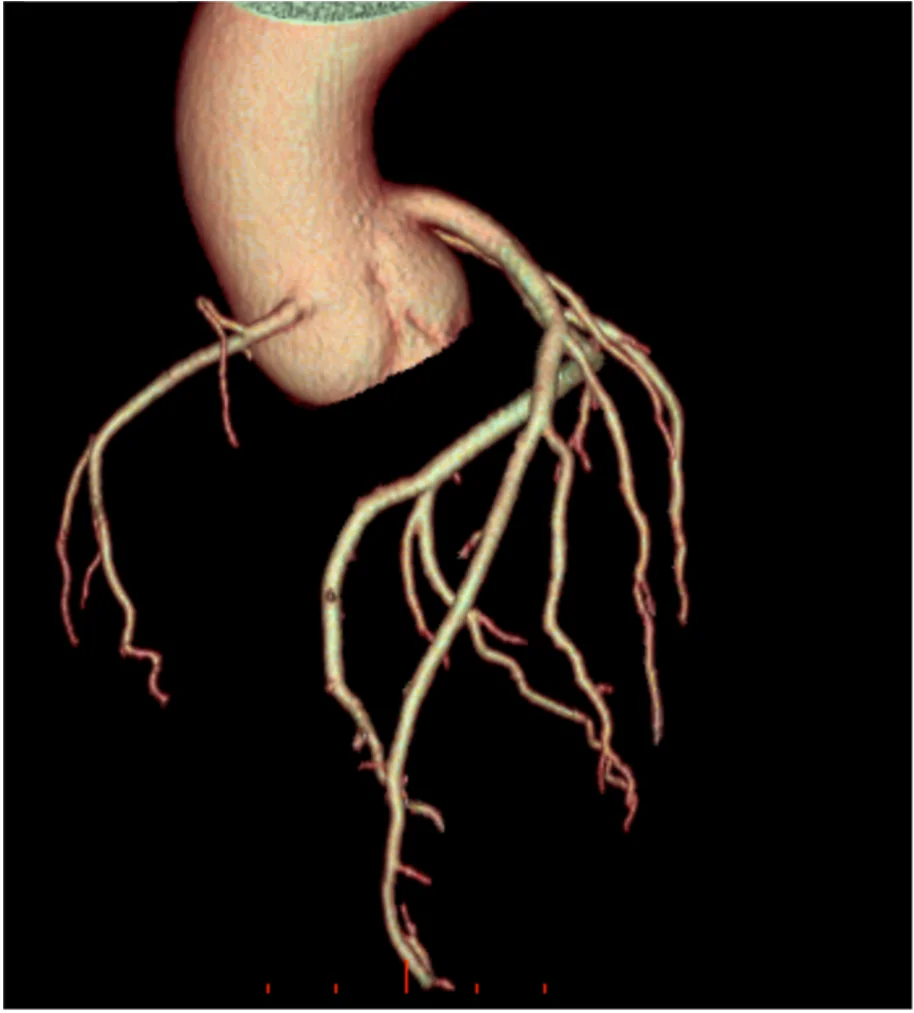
Coronary computed tomography angiography (CCTA) demonstrating epicardial coronary arteries free from significant stenosis at 1‐year follow‐up after the acute event. [Color figure can be viewed at wileyonlinelibrary.com]
